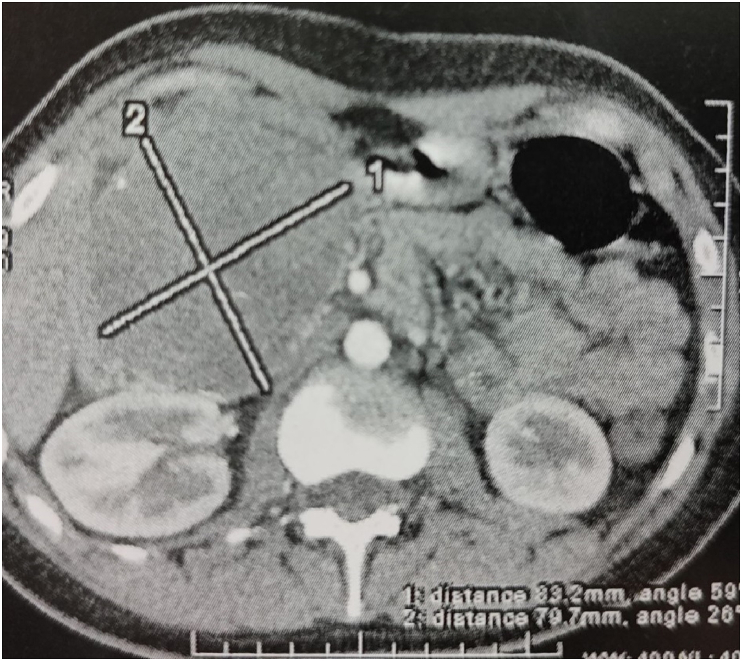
Abdominal CT demonstrates a well-defined mass in the head of the pancreas.
